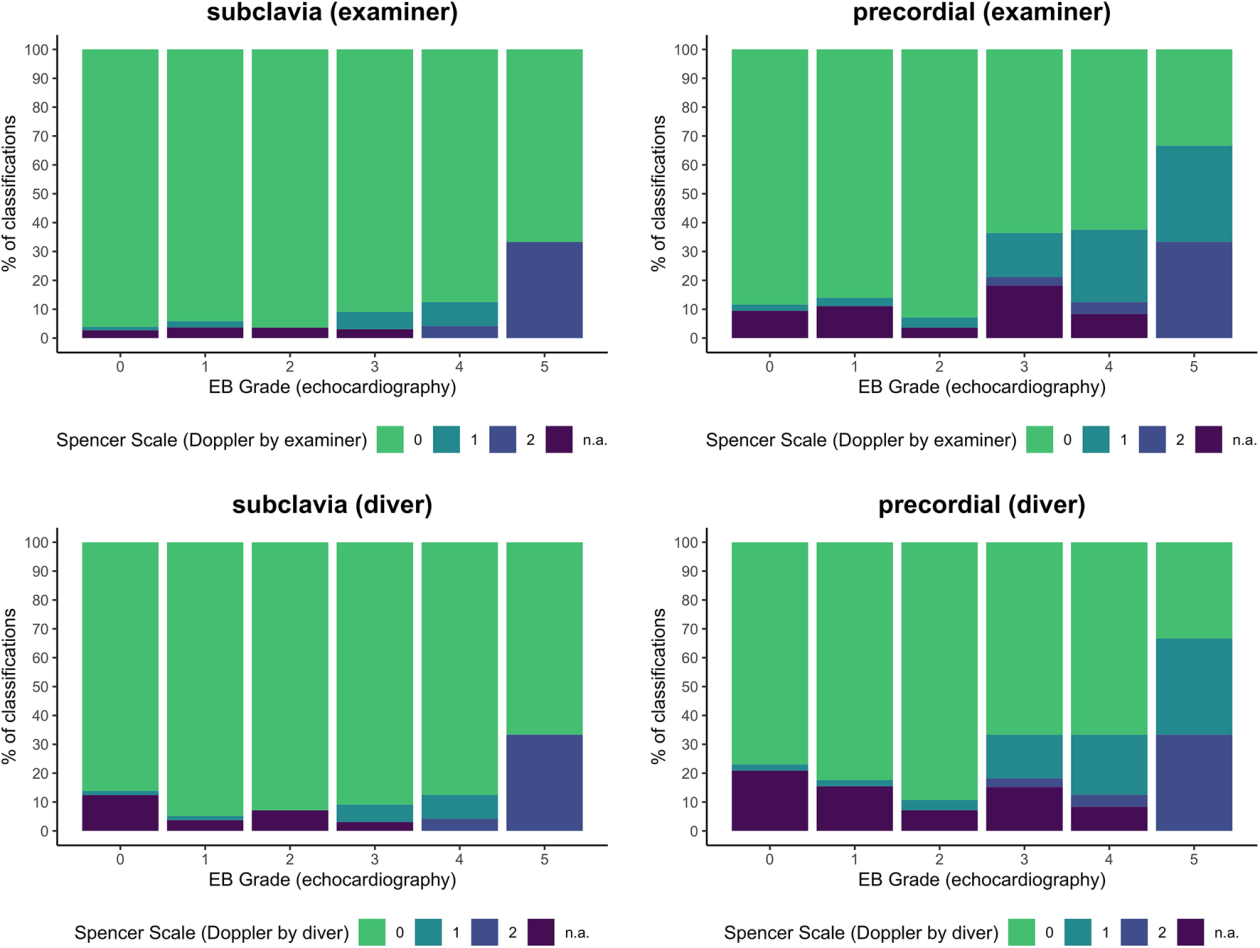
Echocardiography (EB grade) vs. Doppler measurements (Spencer grade) from reference measurements of an experienced medical professional (above) and the trained diver (below). Doppler associations to echocardiographic reference measurements are shown by percentage of audio Doppler detection (Spencer grade, examiner) of 100% of the respective EB grade number
